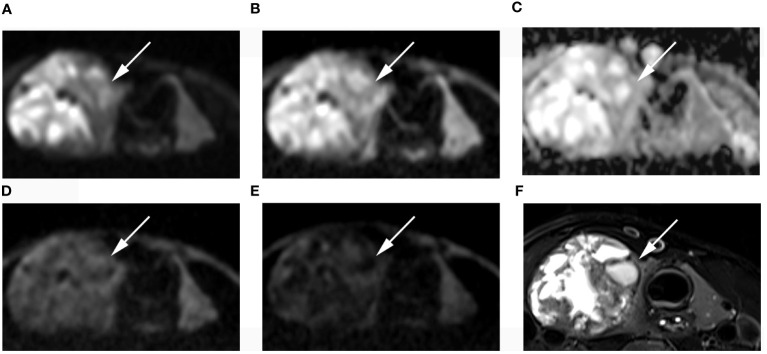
Images of a 49-year-old female with right lobe follicular adenoma (arrow): DWI image with a b-value of 500 s/mm^2^
